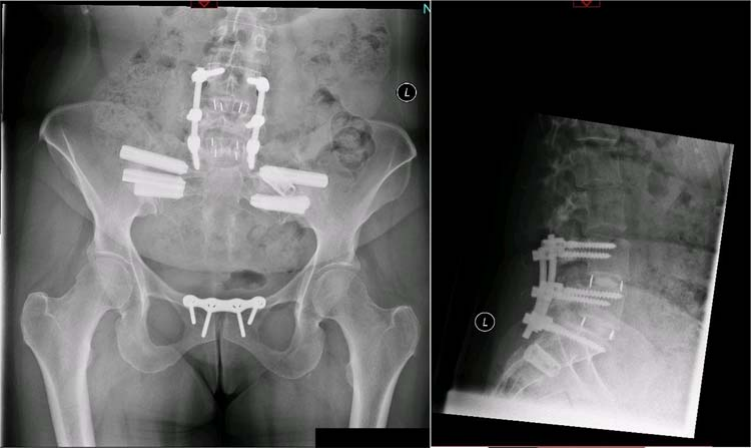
Postoperative X-ray of the pelvis following the third fusion surgery: postoperative X-ray of the pelvis, performed in March 2021 following the third fusion surgery, showing refusion of the left sacroiliac joint and primary fusion of the right sacroiliac joint with the SI-bone implant.
